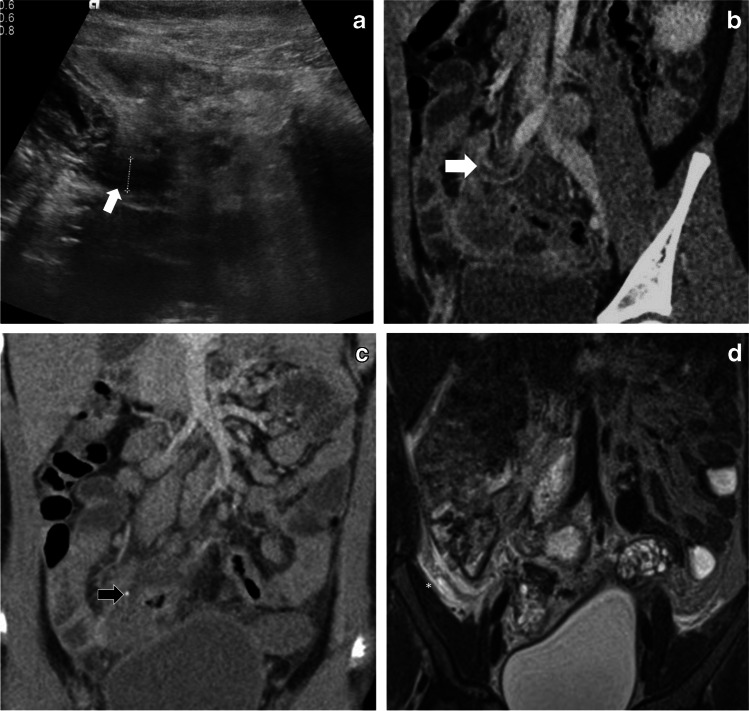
Case 5: A 7-year-old girl presenting with a 3-day history of right iliac fossa pain. a Abdominal US transverse section at presentation shows diffuse right iliac fossa inflammation with free fluid and possible appendicitis. b, c CT scan (coronal post-contrast) next day confirms extensive right iliac fossa mesenteric inflammation with enlarged lymph nodes, free fluid, thickened appendix (6 mm, *white arrow*), with a small 3-mm appendicolith in the mid segment (*black arrow*) and thickened walls of distal ileum, cecum, proximal ascending colon and sigmoid colon. d MRI (T2-W coronal) on day 5 of admission shows similar findings with a small, localized collection in the peri-cecal region (*) with suspicion of a perforated appendix tip. This was followed up on US (not shown), which confirmed resolution of the collection and inflammatory changes after medical management. No surgical intervention was required
